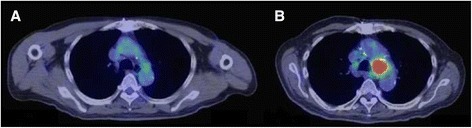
PET/CT depicting increased of FDG uptake at the intrathoracic esophagus site. a PET/CT in 2006 showed no accumulation of FDG around the middle mediastinum. b PET/CT in 2012 indicated FDG uptake on the thoracic esophagus and adjacent lymph node
